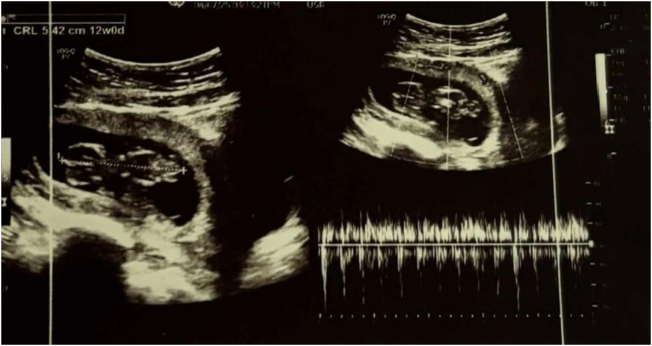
Obstetric ultrasound measuring crown–rump length (CRL).
